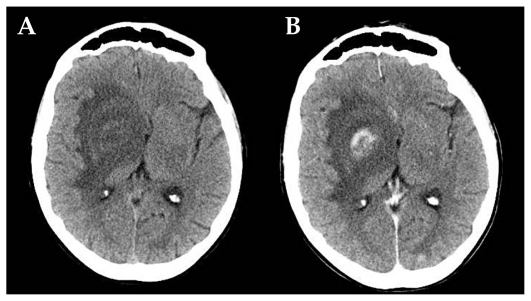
AIDS-related neuro-toxoplasmosis: CT scan of a toxoplasmic localization in the right capsulonuclear region with wide peri-lesional edema before (A) and after (B) contrast injection.
